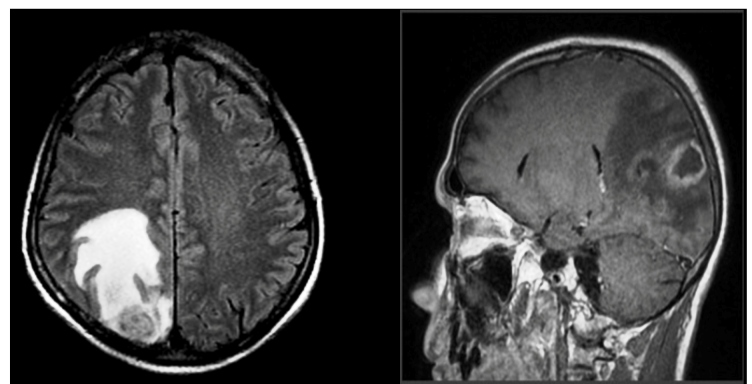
Brain magnetic resonance imaging of the patient shows a right parieto-occipital cortico-subcortical lesion with central necrosis areas and peripherally enhanced area surrounded by perilesional hyperintensity in fluid-attenuated inversion recovery and T2-weighted imaging.
